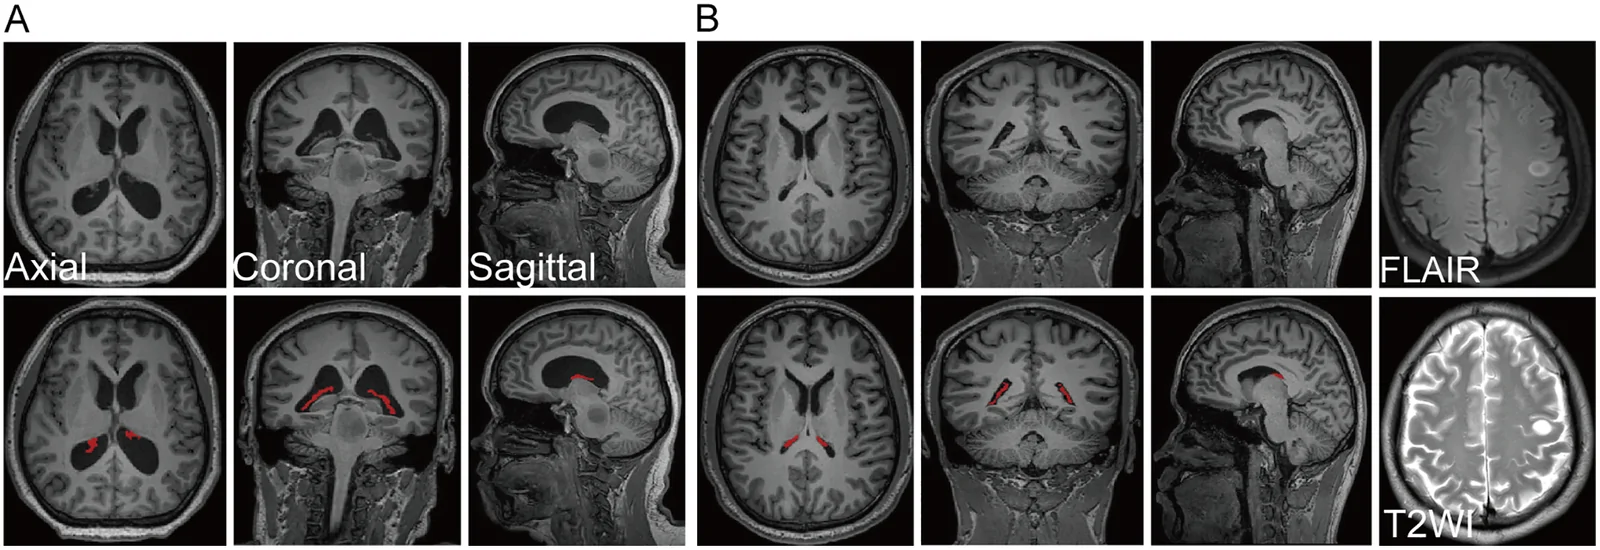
Segmentation of the choroid plexus (ChP) on 3.0 T brain MRI. Representative examples of the ChP (red) in patients with tumors at different locations. (A) A 34-year-old patient with a diffuse midline glioma located in the brainstem. (B) A 34-year-old patient with an astrocytoma located in the supratentorial frontal lobe. The ChP volume is notably larger in the patient with the infratentorial tumor compared with the patient with the supratentorial tumor.
